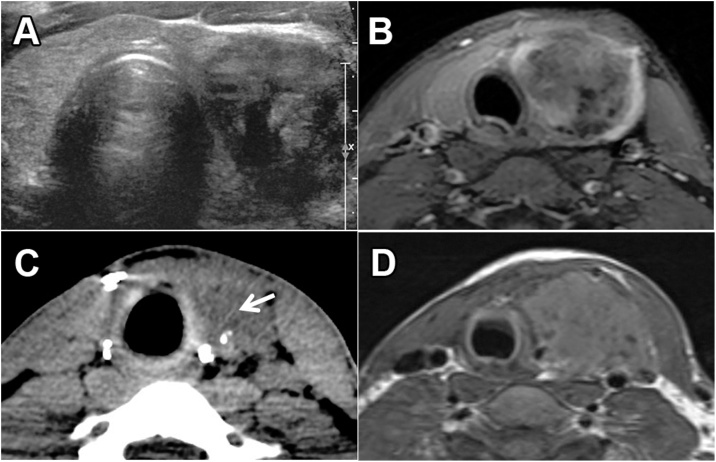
(A) Neck ultrasound revealing a 3.2 × 2.7 × 1.9 cm heterogenous nodule in the left thyroid lobe. (B) Neck MRI performed before left hemithyroidectomy showing no evidence of invasion of the left thyroid tumor into surrounding tissues. (C) Neck CT performed 3 months after completion thyroidectomy revealing a 2 cm fluid accumulation in the left thyroid bed (arrow) but no evidence of tumor recurrence. (D) Neck MRI revealing a 10.5 cm tumor in the left thyroid bed 16 months after the initial left hemithyroidectomy.
